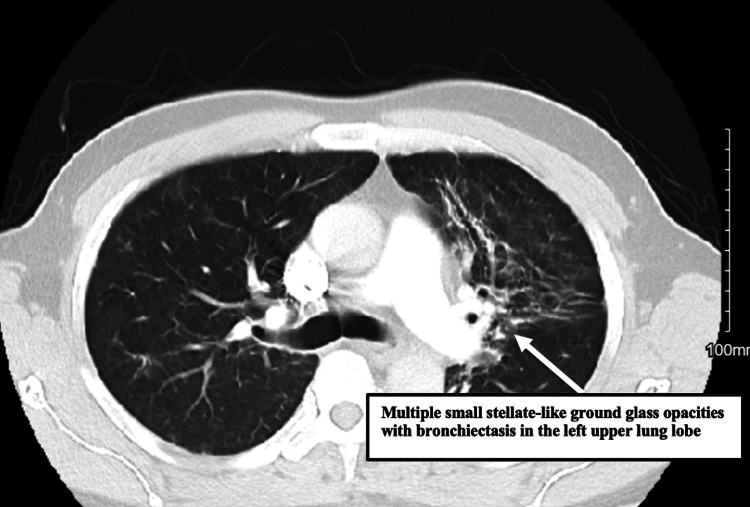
CT pulmonary angiography showing multiple small stellate-like ground glass opacities with bronchiectasis in the left upper lung lobe. CT: computed tomography
